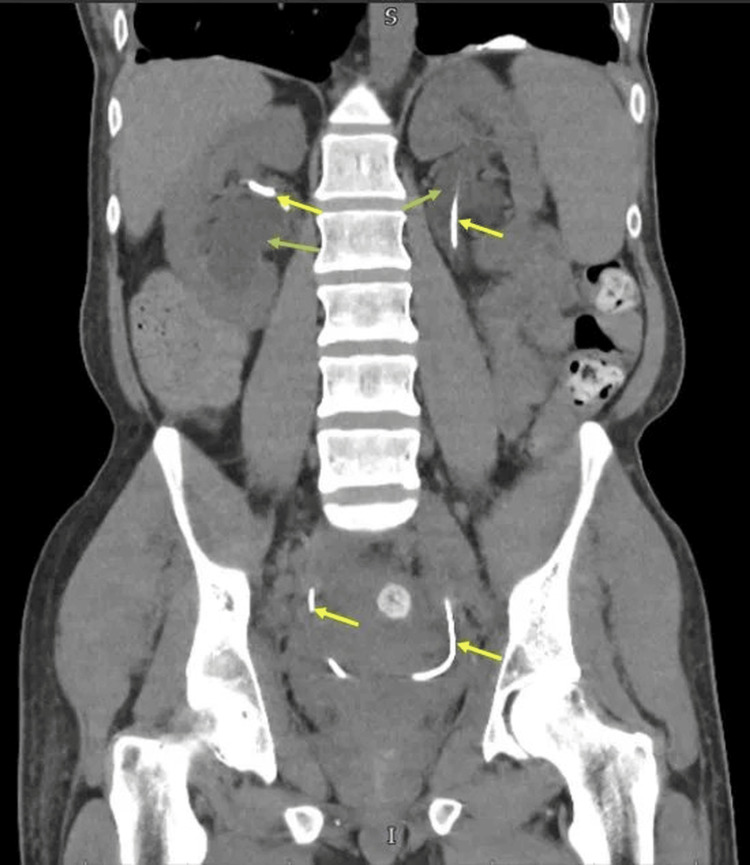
Coronal non-contrast CT of the urinary tract performed post bilateral JJ stent insertion Both stents are seen in satisfactory positions (yellow arrows). Despite appropriately sited stents, bilateral hydronephrosis and pelvicalyceal dilatation persist (green arrows), with slight worsening on the right when compared with previous imaging. This is consistent with extrinsic ureteric obstruction rather than an intrinsic cause that is amenable to stenting.
